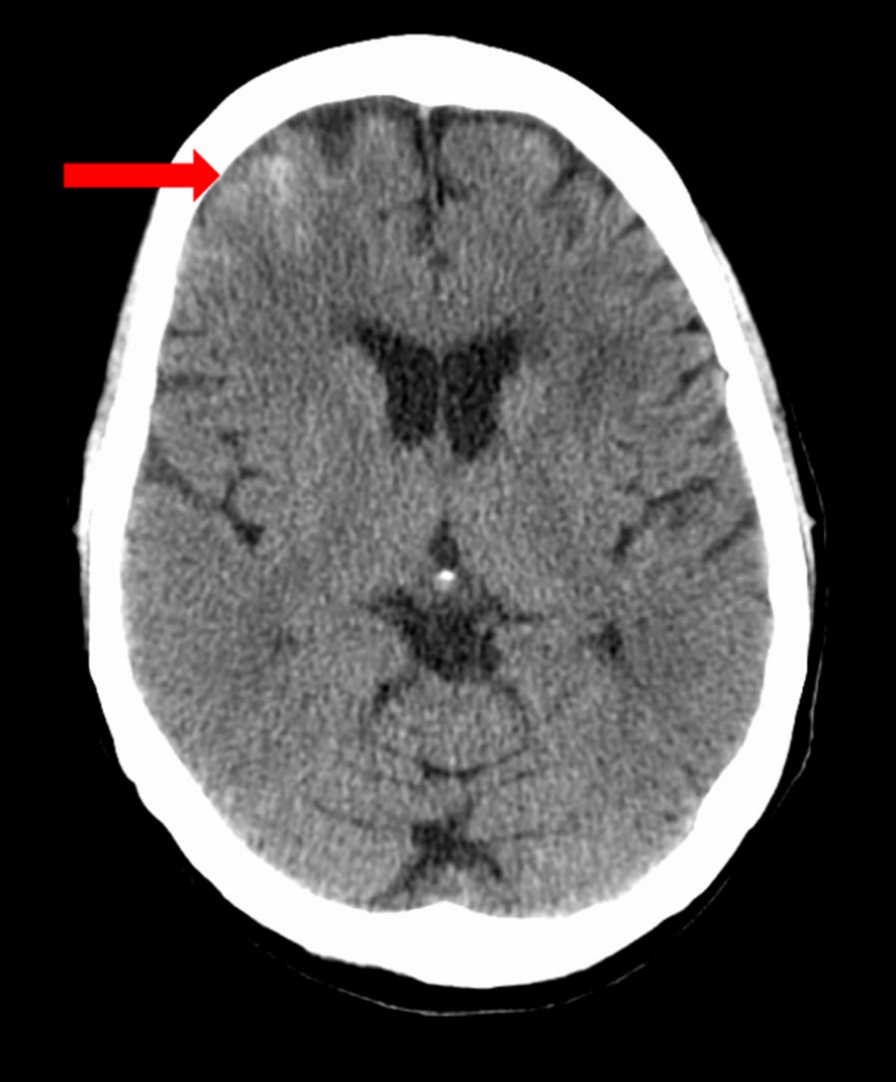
CT scan of the brain (axial sequence) shows an area of hyperdensity in the right frontal lobe suggestive of intracerebral hemorrhage (*arrow*) in a 67 year old woman who underwent right carotid endarterectomy (CEA) for the treatment of a 95 %* right* ICA stenosis. The patient post-operatively developed headache, photophobia and intermittent dizziness. This CT brain was done almost 24 h after the CEA. Systolic blood pressure was in the 170 s mm Hg and difficult to control as after CEA there was thought to be a clamp injury to the right carotid artery bulb. However, the patient did well clinically and at her 3 month follow up office visit, she had no residual neurological deficits
